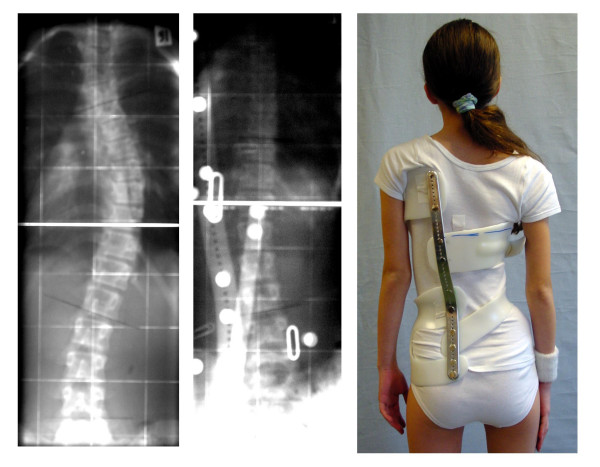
Example of a patient with an overcorrection in a Chêneau light brace. Overcorrection of a thoracic curve from 38° to -14° in a T2 „Chêneau light" model in an 11-year old premenstrual girl with Tanner II. For the measurement of the corrected curve the neutral vertebra of this X-ray was used. If we would have taken the neutral vertebrae of the previous X-ray the correction effect would have been less important.
